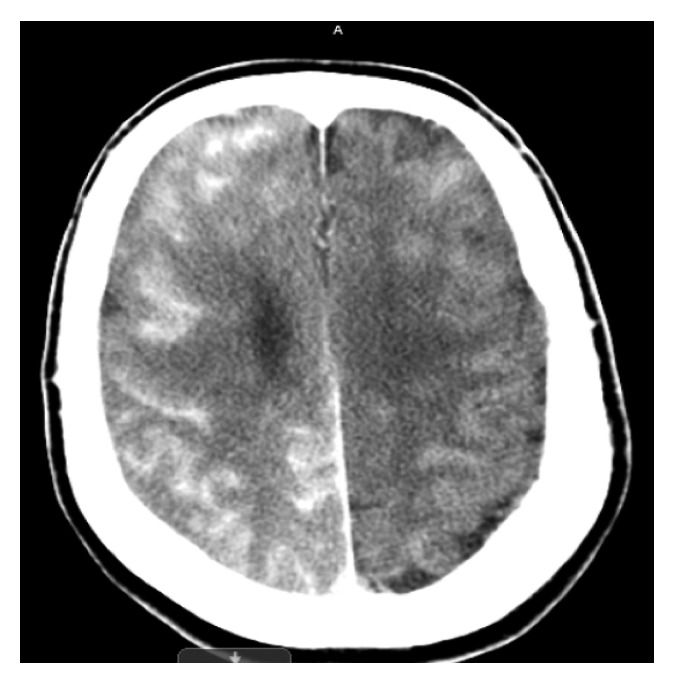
CT brain showing increased hyperdensity and enhancement of right cortex.
